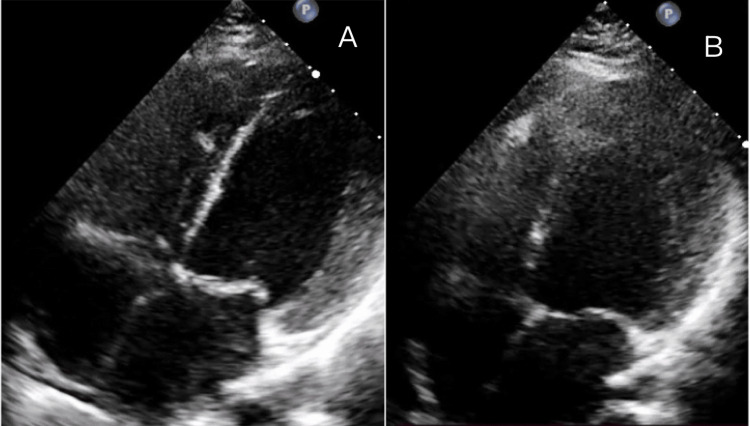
Apical four-chamber view (A) at diagnosis suggesting dilated left ventricular cavity. LVEF was diagnosed using the modified Simpson’s method. (B) At the one-month follow-up. LVEF = left ventricular ejection fraction
